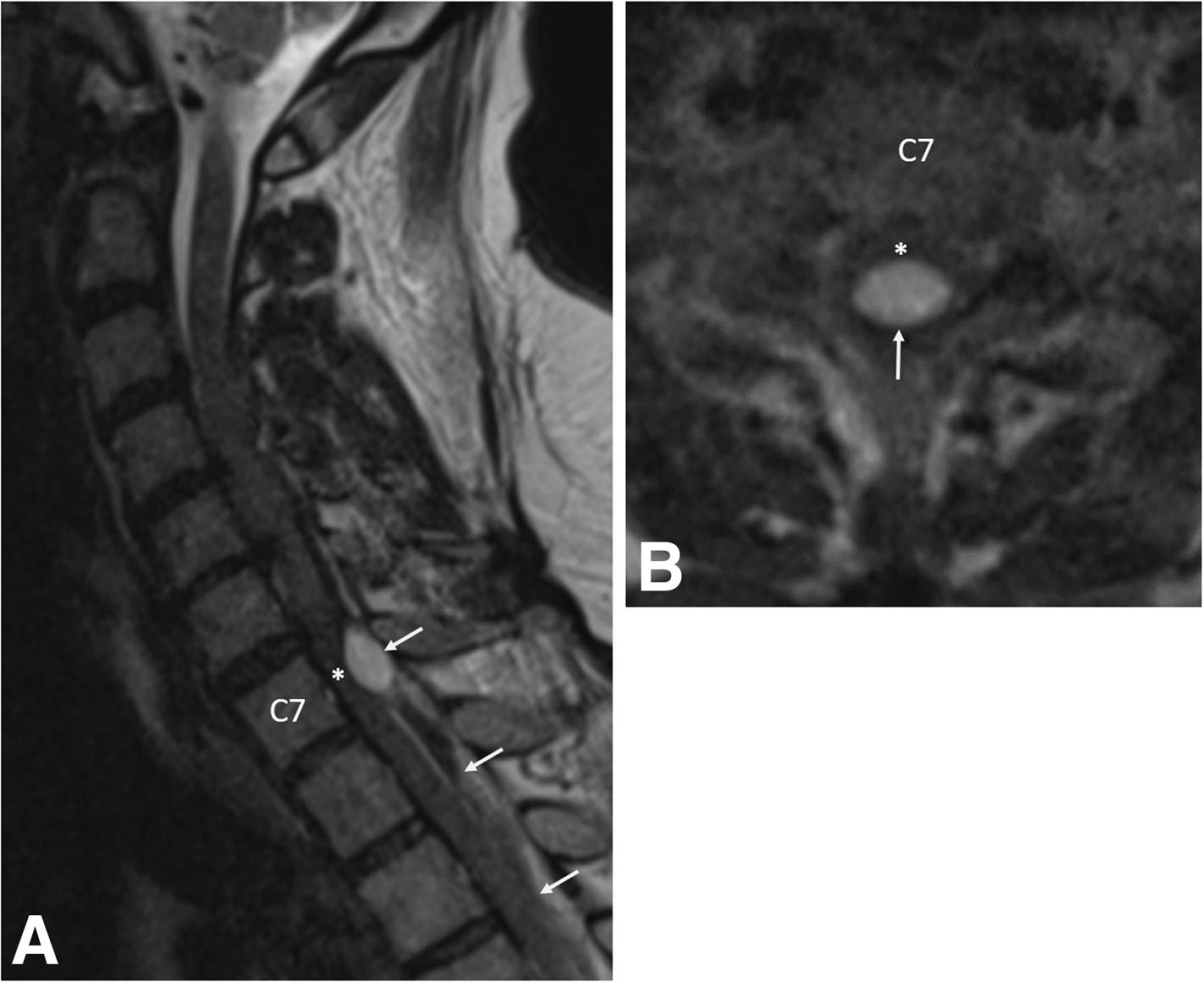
a Pre-operative sagittal T2-weighted MRI of the cervical spine demonstrates an epidural hematoma (arrows) with the cranial extent at the C6/7 level. The hematoma is mixed signal intensity, consistent with various stages of bleeding. Severe spinal cord (asterisk) compression is present at the C7 level. b Pre-operative axial T2-weighted MRI at the C7 level demonstrates a loculated epidural hematoma (arrow) compressing the spinal cord (asterisk). Due to the urgent nature of the MRI, image quality is degraded
